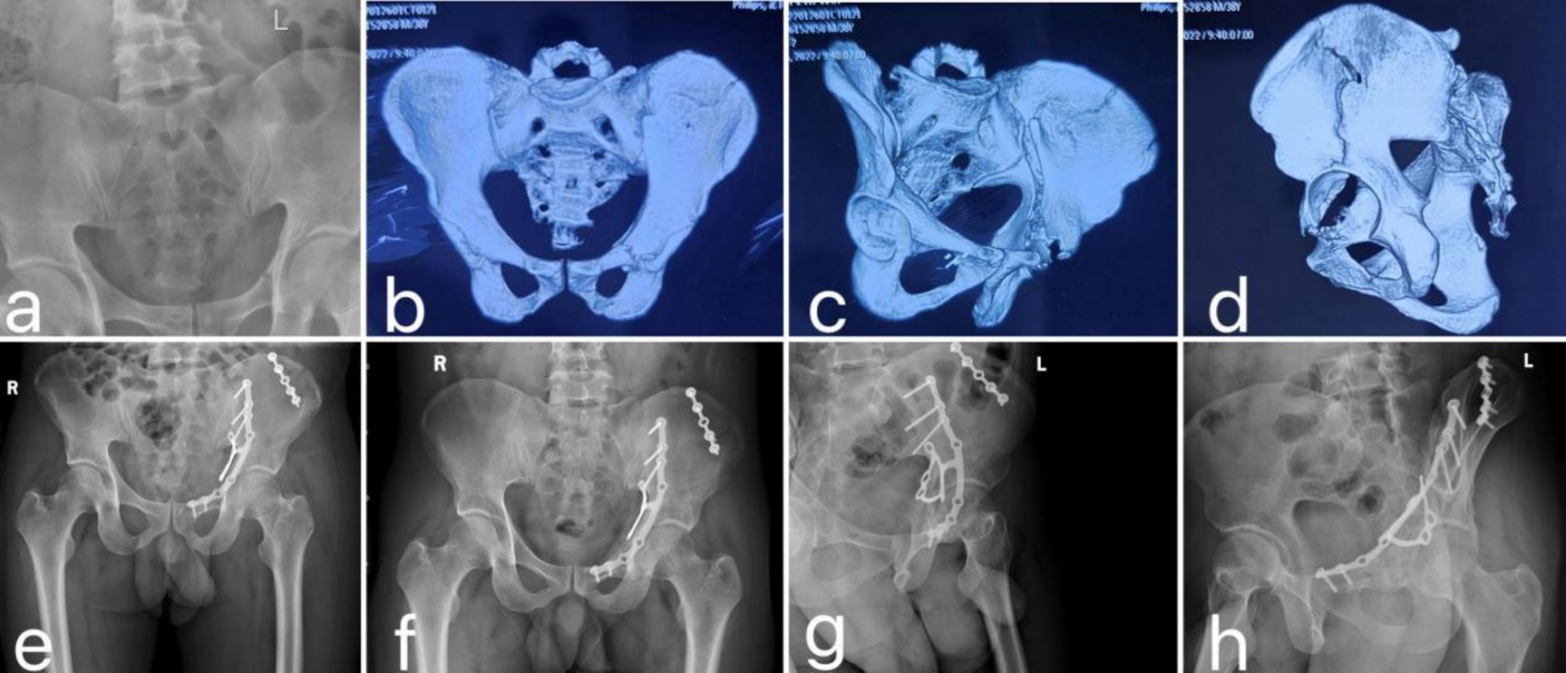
Typical Case 1: A 38-year-old male patient with a T-type acetabular fracture undergoing surgery using 3D printing technology combined with customized steel plates. a: Preoperative pelvic X-ray. b-d: Preoperative pelvic CT scan with three-dimensional reconstruction examination. e: Pelvic X-ray on the first day postoperatively. f-h: Pelvic X-rays at 12-month follow-up (including anteroposterior view, pelvic outlet oblique view, and iliac oblique view).
